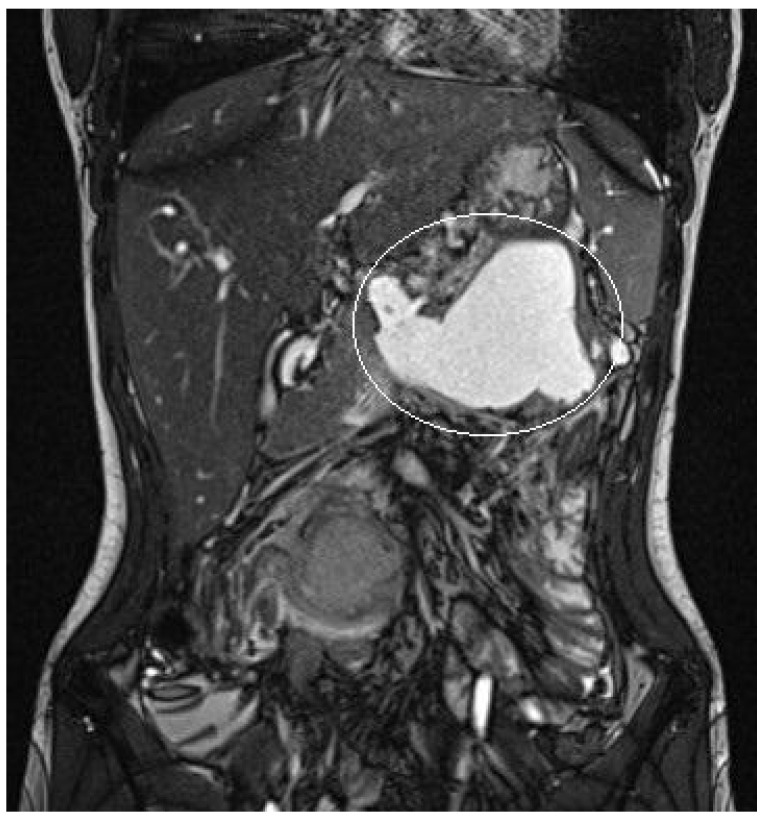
Patient n°1 MRI. Pseudocyst in the omental bursa with maximum diameter 10 cm.
